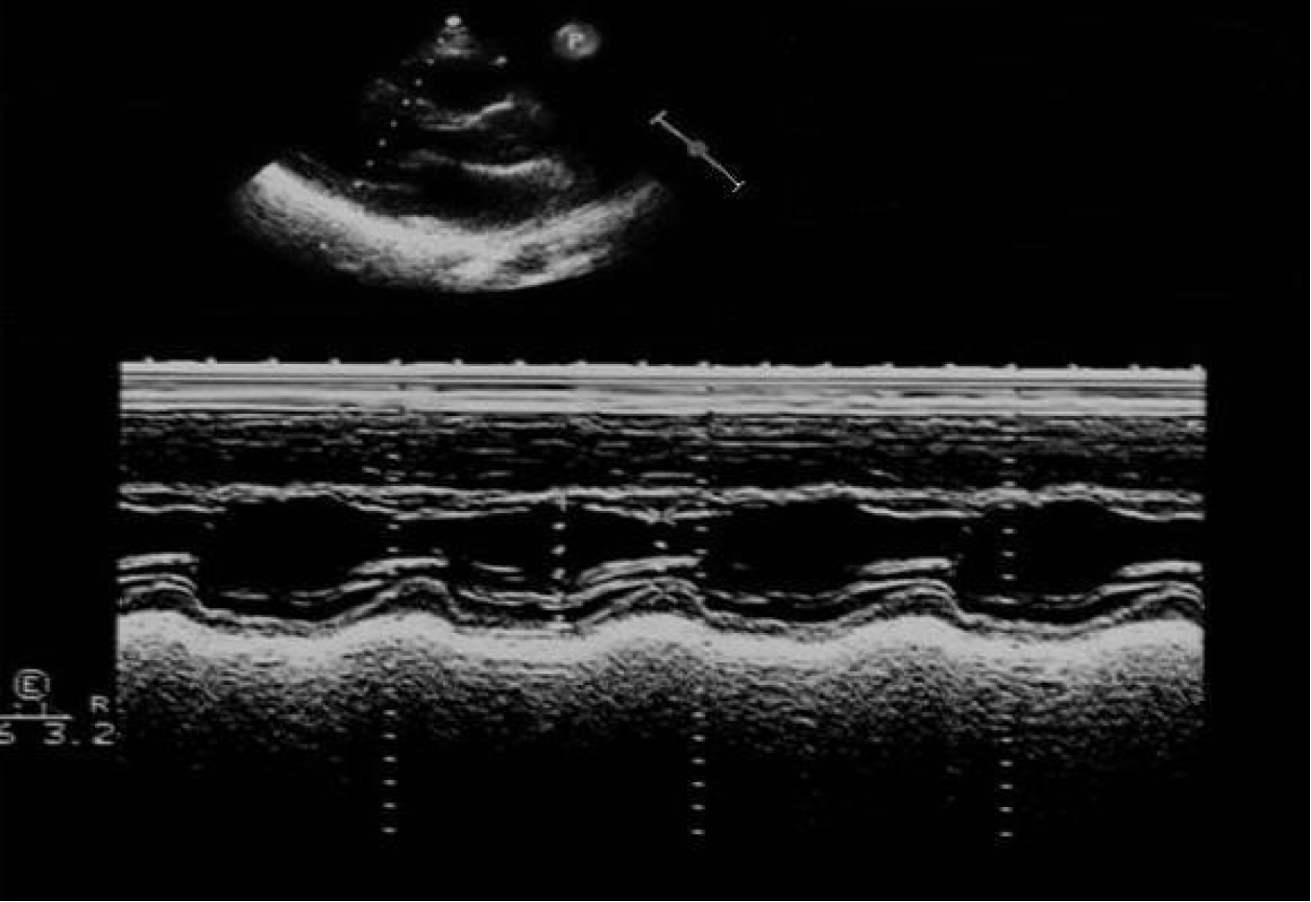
Transthoracic echocardiogram: parasternal log axis view showing septal paradoxical movement at M-mode modality.
